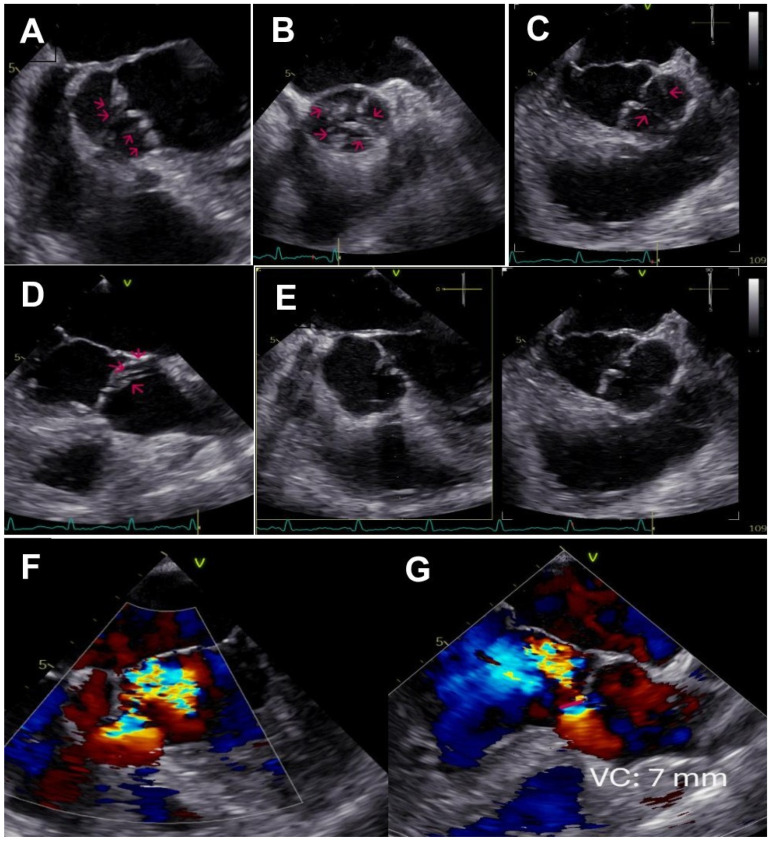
To better quantify the degree and the mechanisms of aortic regurgitation, the patient underwent transesophageal echocardiography (TEE), which revealed multiple chordae tendineae strands (ACTS) originating from the sinuses of Valsalva and inserting into the aortic cusps, as shown by red arrows in several mid-esophageal views (0°, panel (A); 45°, panel (B); 90°, panel (C); 113°, panel (D); Supplementary Video S2). These chordae exert asymmetric traction during diastole, leading to incomplete cusp coaptation (X plane 0–90° mid-esophageal view, panel (E)) with type III aortic regurgitation according to the Carpentier classification, with a severe holodiastolic eccentric jet (VC 7 mm) directed posteriorly toward the AML (mid-esophageal 0°, panel (F); mid-esophageal 132°, panel (G); Supplementary Video S2). The occurrence of ACTS is rarely documented in the literature, and the genesis remains unclear. However, it has been hypothesized that they represent vestigial embryonic remnants arising during the morphogenesis of the aortic valve cusps [2]. This condition predominantly affects middle-aged Asian males with a tricuspid aortic valve morphology [3].
